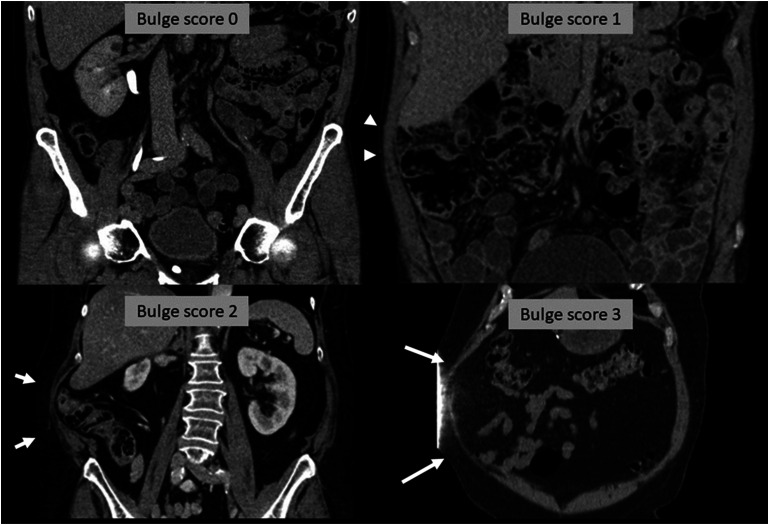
Coronal reformatted CT images showing examples of the different scores of abdominal bulge. The example for bulge score 0 was treated on the left side (right in image) while the other examples were treated on the right side (left in image). Bulge score 1 (arrowheads) with local thinning of right lateral abdominal muscles. Bulge score 2 (short arrows) with severe local bulging of the right lateral abdominal muscles. Bulge score 3 (long arrows) with severe thinning and asymmetry of the right anterolateral abdominal muscles.
